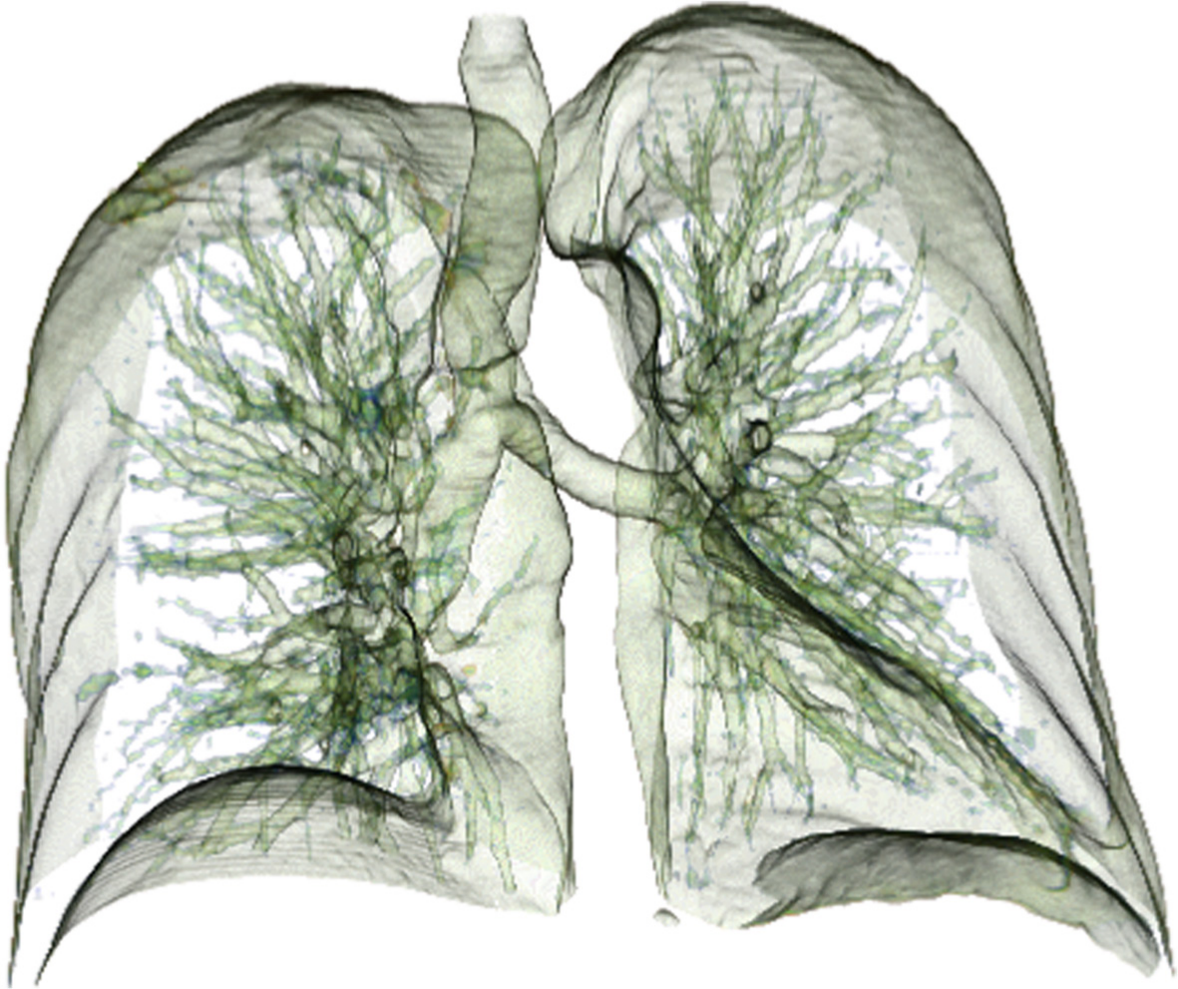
Image of lungs segmented through the 3D Slicer tool. Using EM Segmentation algorithm to separate the lung region from other organs and tissues on the computed tomography image with 3D slicer tools.
